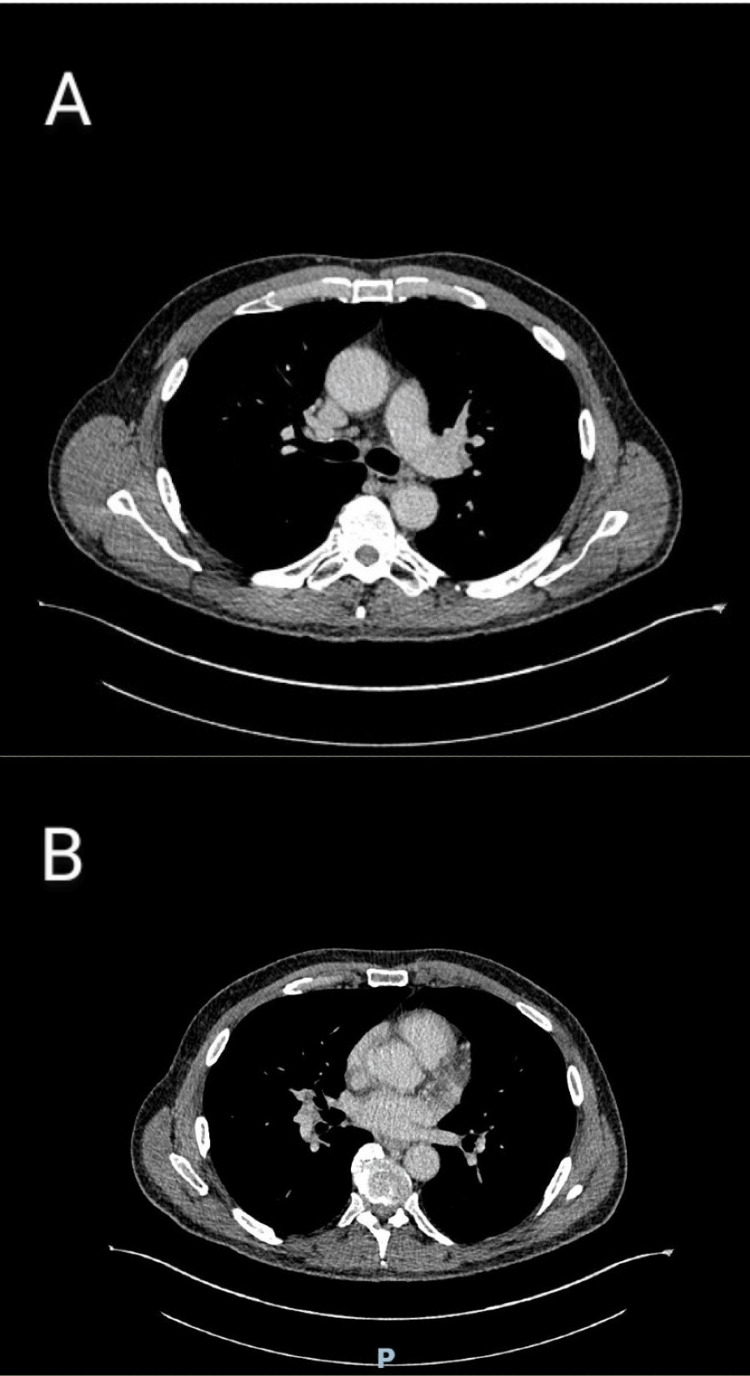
Outpatient CT scan showing resolution of the hilar and mediastinal lymphadenopathy Comparing this image with the previous images, you can see that the panel A shows the hilar region without the enlarged lymph nodes seen in Figure 2 while panel B shows the mediastinal region without the previously seen enlarged lymph nodes in Figure 2.
